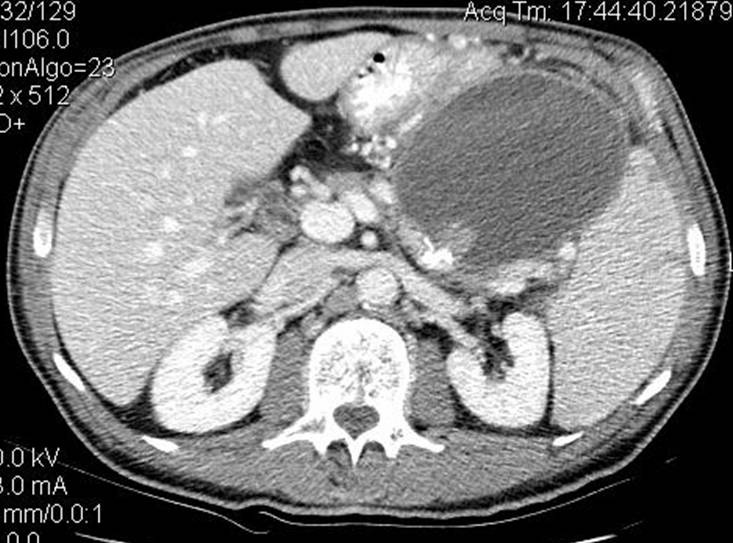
Venous phase CT shows a dubious new finding suggesting a minimal vascular abnormality within the pseudocyst.
